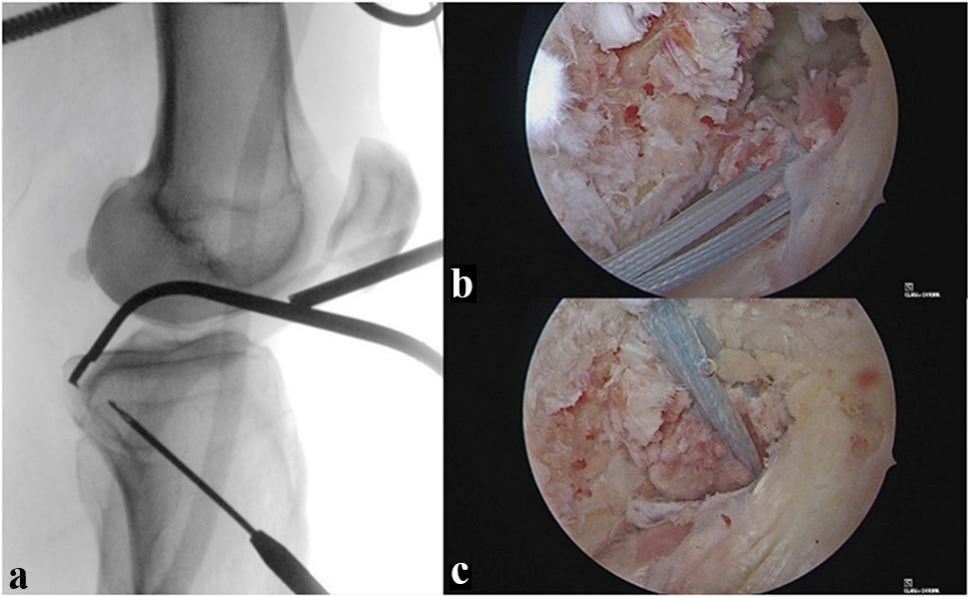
Intraoperative radiographic control of tibial tunnel placement for PCL bracing (a). Intraarticular, arthroscopic view with PCL pullout sutures in-situ (b) and reattached femoral PCL avulsion with ligament bracing (c). *PCL* posterior cruciate ligament
